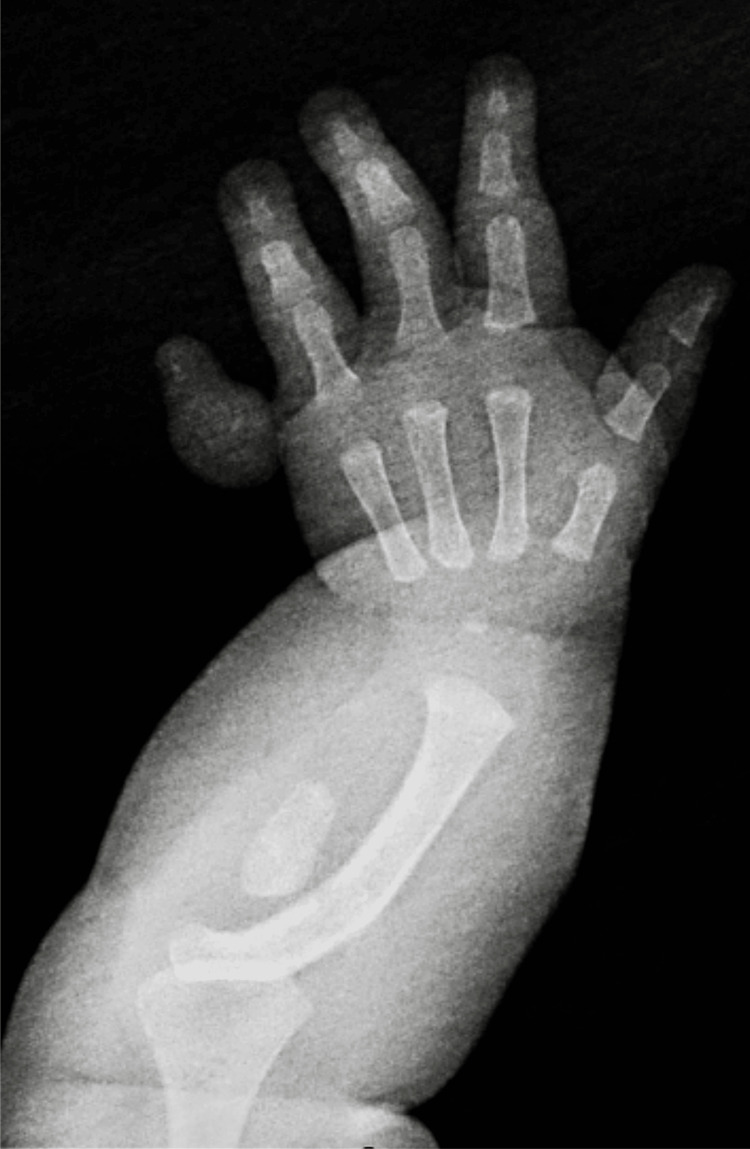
Left forearm and hand radiograph depicting a small ovoid bone in the expected location of the ulna without articulation to the humerus or wrist.
